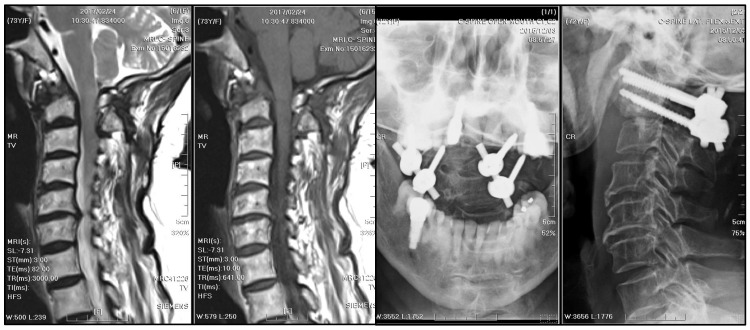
MRI and radiographs of cervical spine after antitubercular treatment: A 12-month antitubercular treatment regimen led to significant neurological improvement and a substantial reduction in the size of the mass, which became nearly undetectable on subsequent MRI. The stabilization remained fair.
